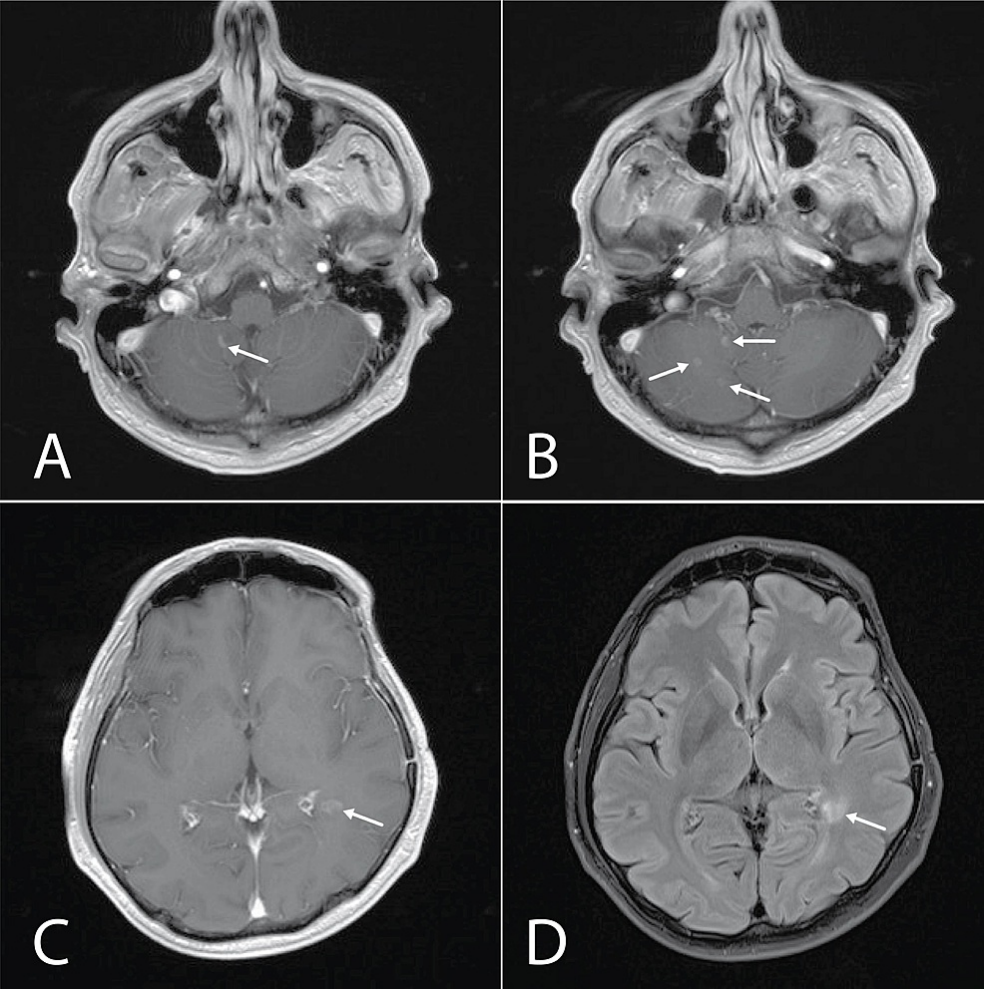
Brain MRI with IVC. A. hyperintense open ring-type non-homogeneous contrast enhancement of cerebellar lesion (arrow) (T1 post-contrast sequence); B. hyperintense non-homogeneous contrast enhancement of cerebellar lesions (arrows) (T1 post-contrast sequence); C. hyperintense open ring-type non-homogeneous contrast enhancement of lesion in left periventricular white matter (arrow) (T1 post-contrast sequence); D. left periventricular lesion without perifocal edema (arrow) (T2/FLAIR sequence). IVC: intravenous contrast; FLAIR: fluid-attenuated inversion recovery
